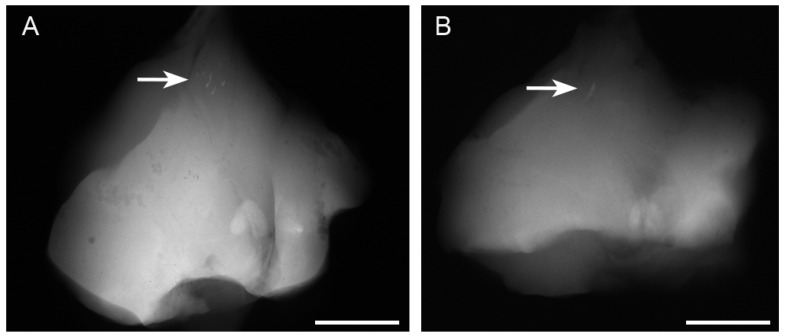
Presence of opacities on soft X-ray films after *bmp-2*-expressing plasmid electroporation. The opacities (white arrow) in the targeted muscles after electroporation with (A) needle- and (B) plate-type electrodes. Several opacities (white arrow) in the skeletal muscle *bmp-2* gene were transferred with needle-type electrodes (A). One opacity (white arrow) was found in the skeletal muscles with plate-type electrodes (B). Each scale bar is 15 mm.
